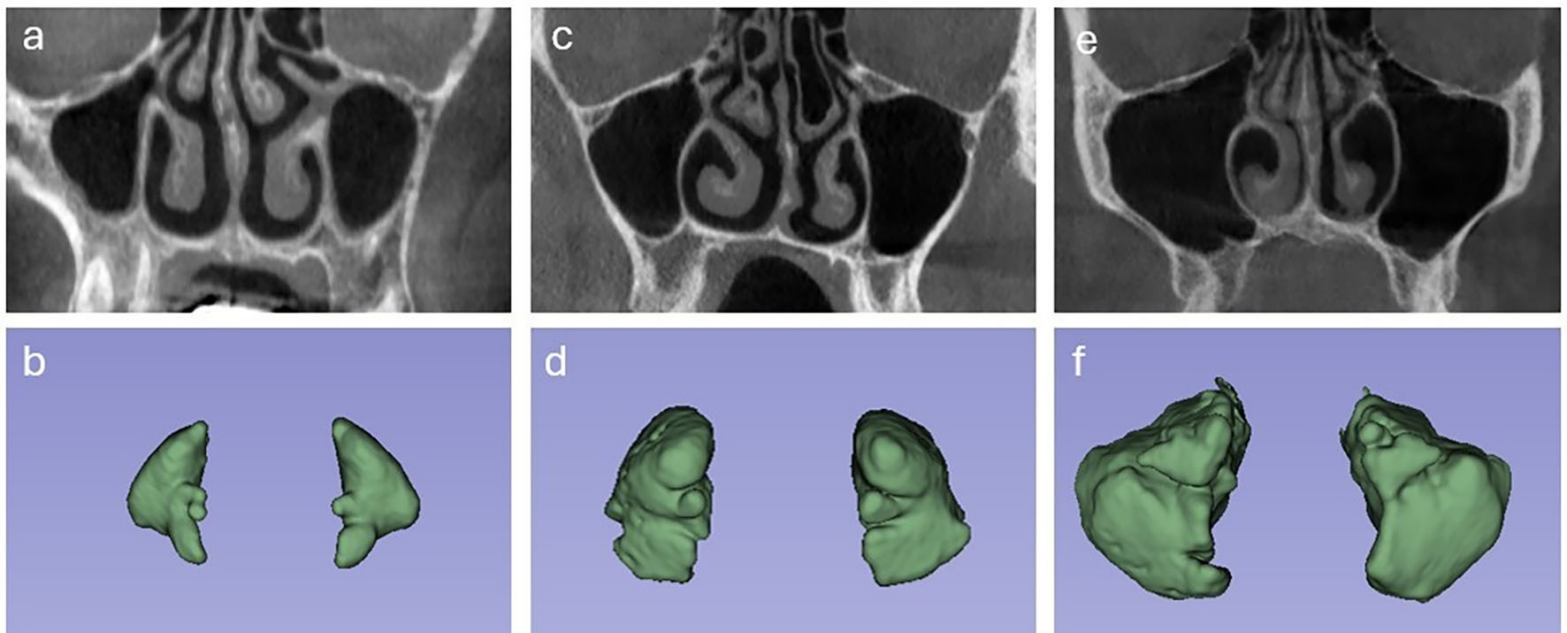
Coronal CBCT image and three-dimensional reconstruction of, a-b hypoplastic maxillary sinus (MS), c-d normal MS, and e-f hyperplastic MS
